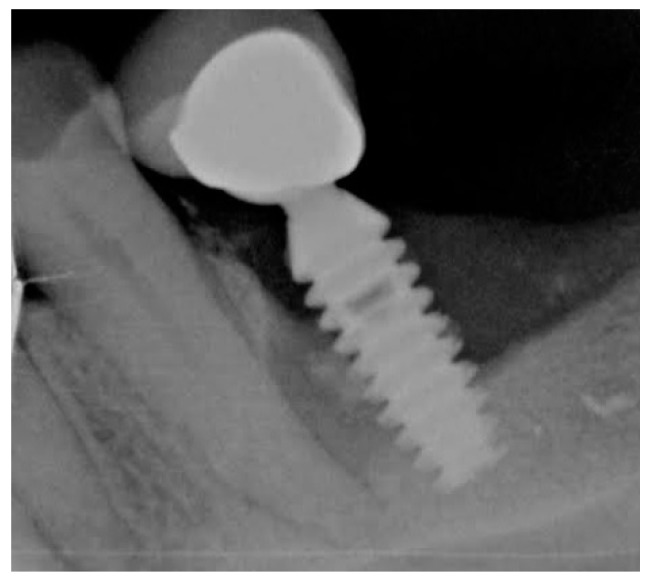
Image obtained during clinical practice of Dr. L. Fiorillo. Radiographic examination of implant with peri-implantitis due to the formation and organization of a biofilm on the implant surface. The interface between the bone tissue and the implant has been interrupted, this must be minimal and in the absence of biofilm to guarantee integration.
